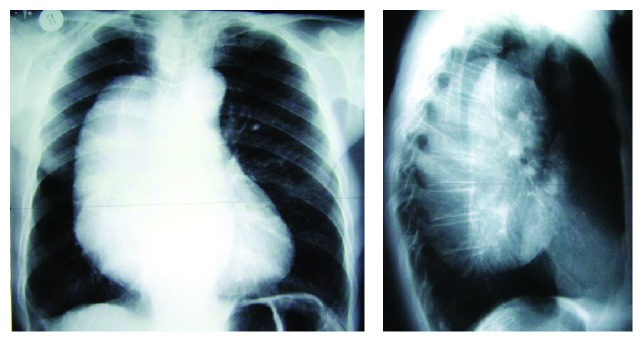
Preoperative chest X-ray showing a large intrathoracic tumor.
